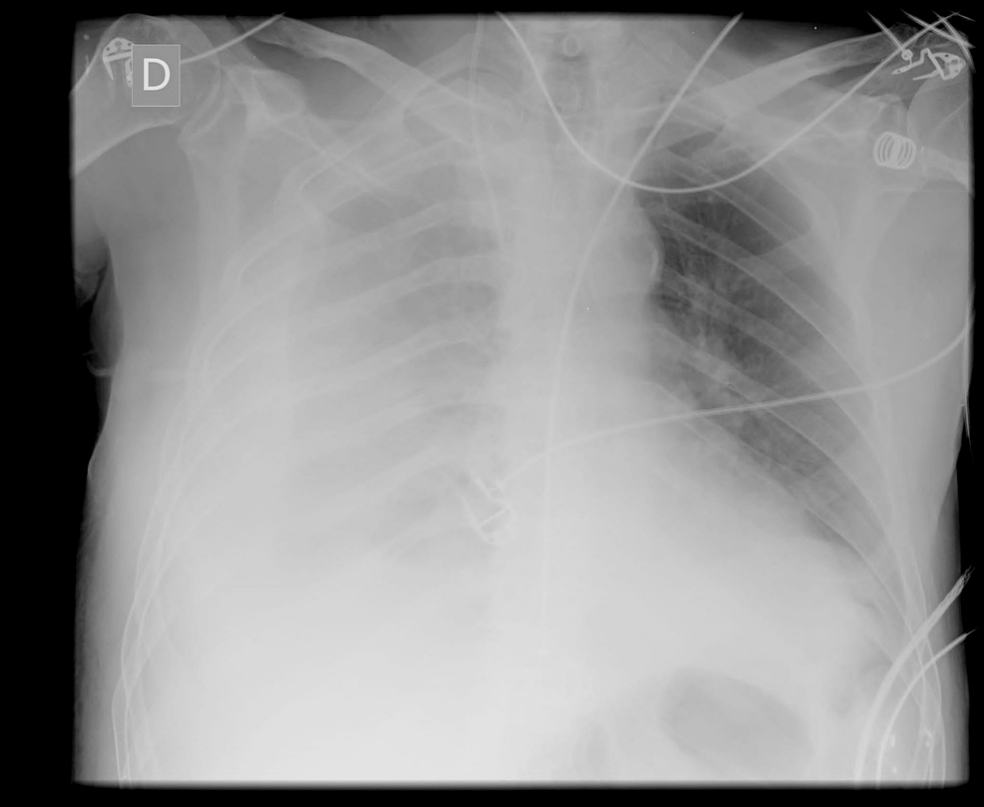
Thoracic radiography 24 hours post block
